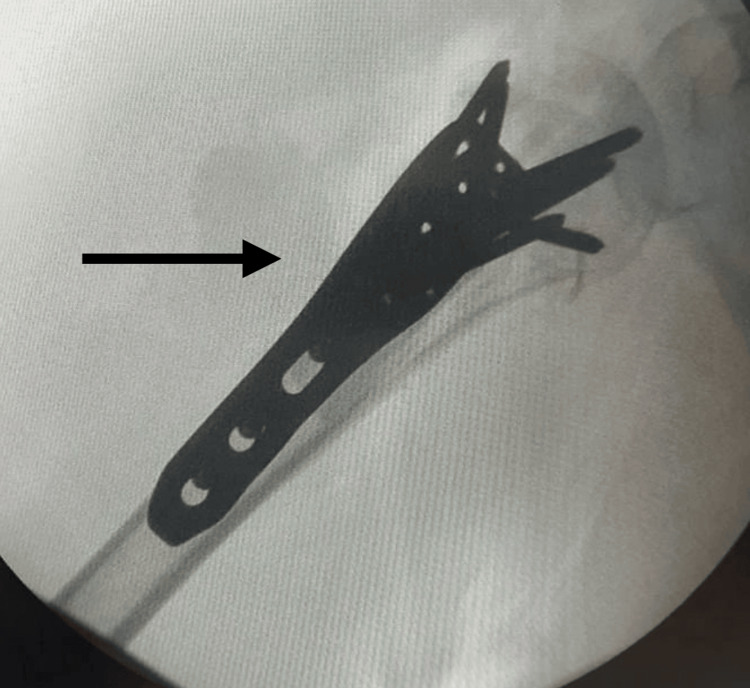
AP X-ray of the right shoulder revealing the right proximal humerus postoperatively The arrow shows the postoperative results: fracture reduction, plate, graft, and screw placement.
